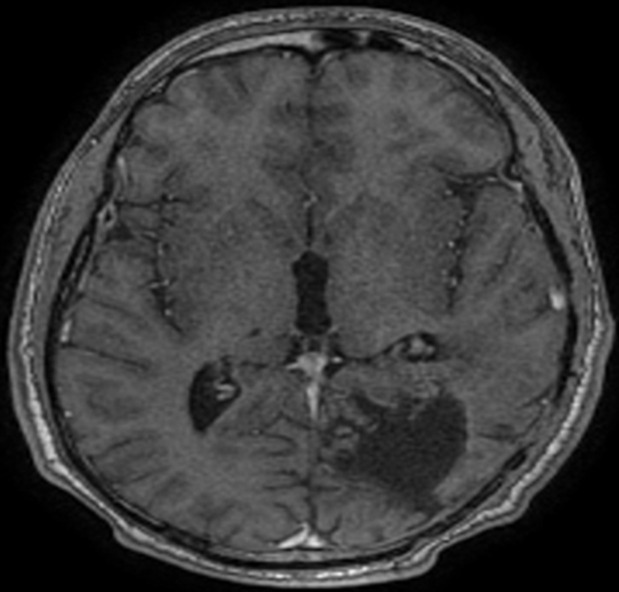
MRI T1, axial section showing the disappearance of the tumor
